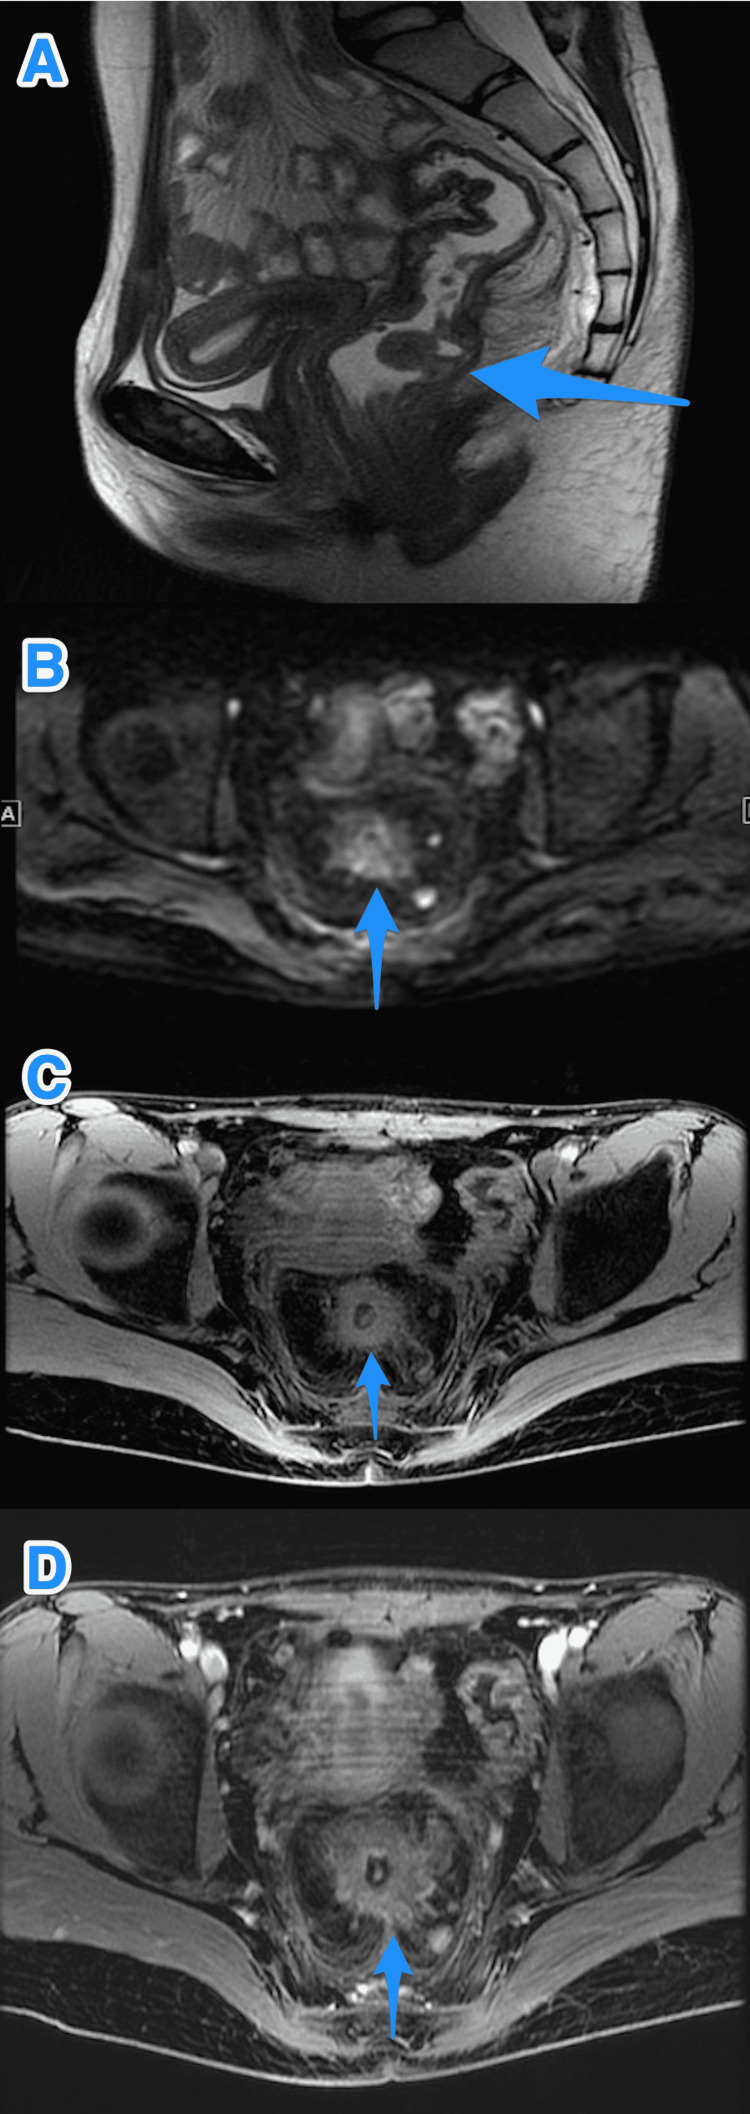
Follow-up (post-chemoradiotherapy) MRI study of the pelvis. MRI study of the pelvis sagittal T2 (A), axial DW (B), axial T1 (C), and axial T1 post-contrast (D) photographs shows significant interval improvement of the mid rectal tumor (small arrow) and mesorectal lymph nodes. The lower rectal polyp is also showing post-treatment response and size regression (large arrow). DW: diffusion-weighted
